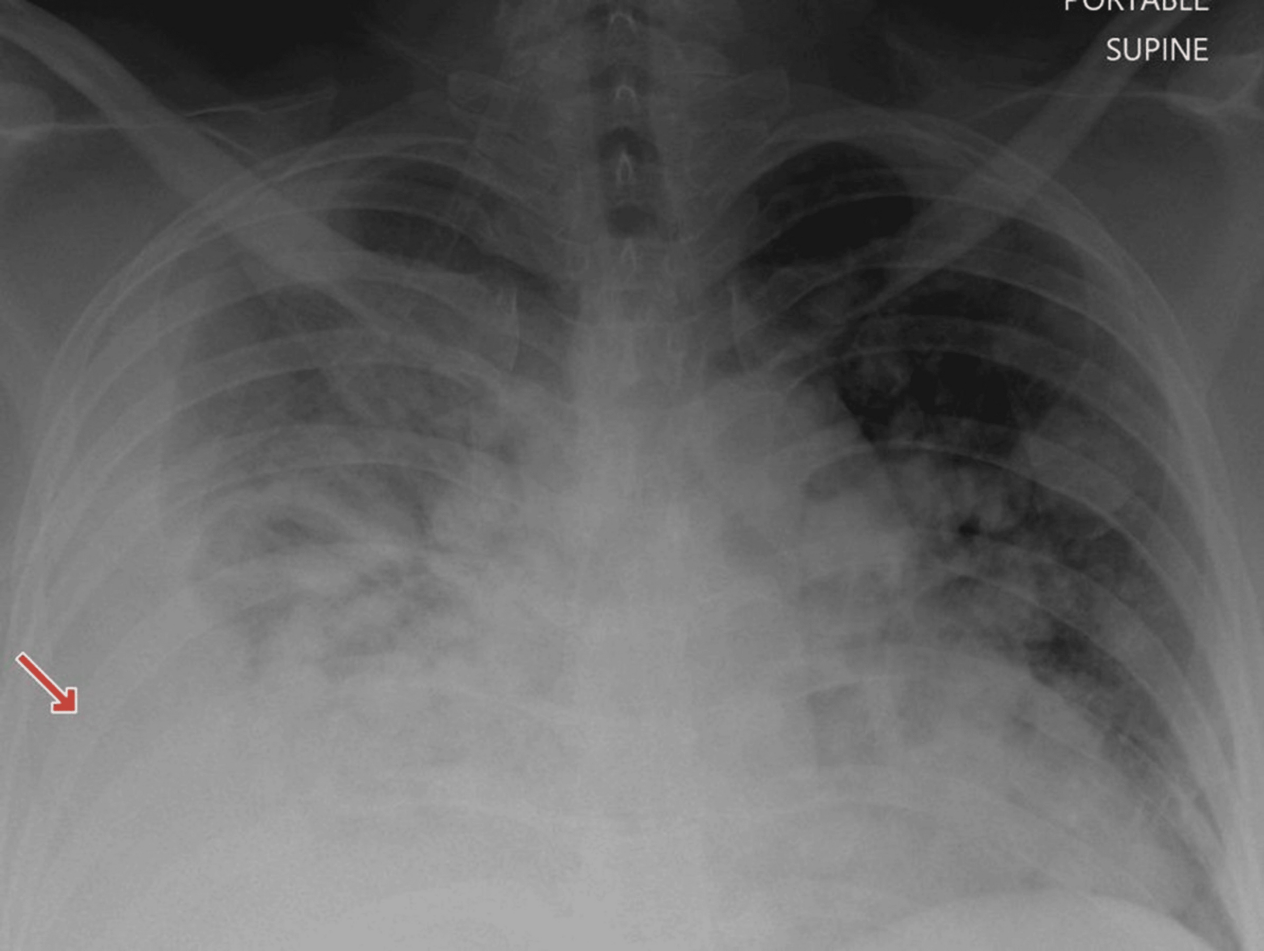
X-ray chest PA view showing interval development of moderate right sided pleural effusion.
